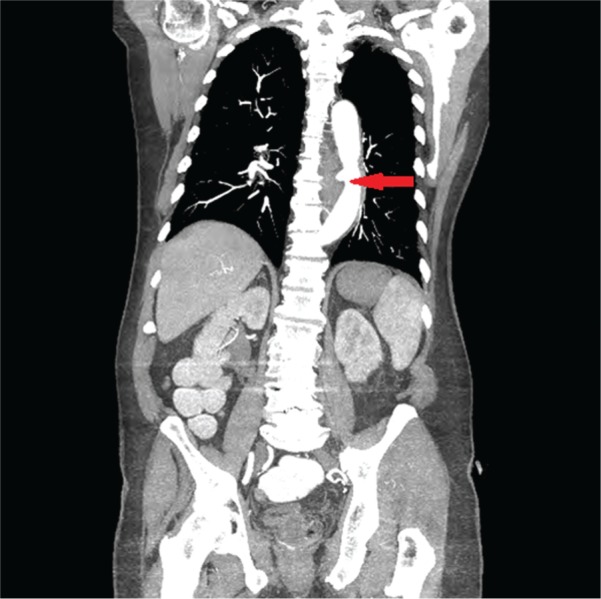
CT angiography of the chest and abdomen (coronal section). The arrow shows out-pouching of the intravenous contrast within the descending thoracic aorta, with marked mural thickening of the adjacent esophagus, with extra luminal foci of air between the esophagus and the aorta.
